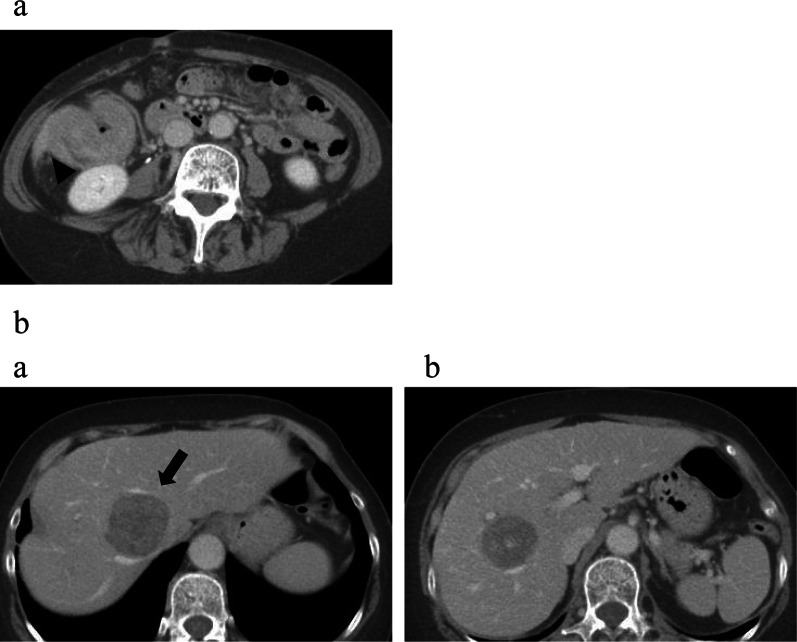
Computed tomography images before preoperative chemotherapy. a An enhanced mass in the ascending colon is seen invading the abdominal wall and the inferior edge of the liver (black arrowhead). b (a) The ventral lesion is seen touching the middle hepatic vein (black arrow). (b) The dorsal lesion of hepatic metastasis
